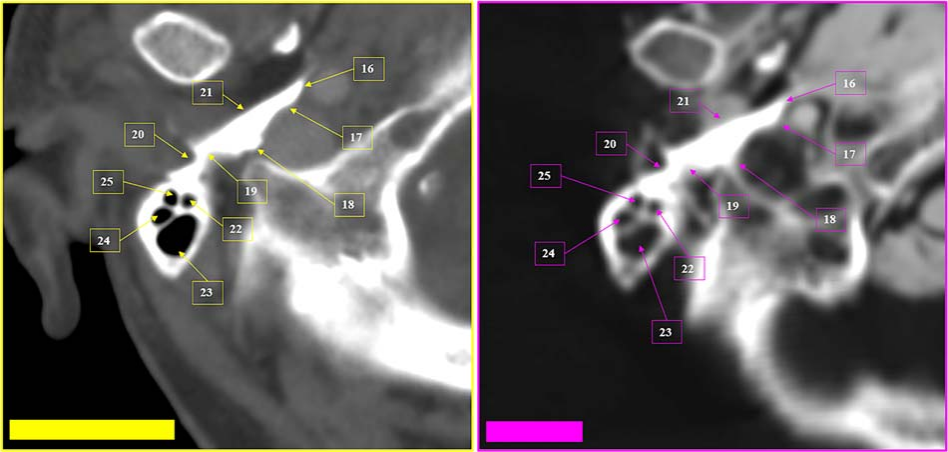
Coinciding morphology details and the arrangement of right mastoid cells in the antemortem (left) and postmortem (right) CT scans in axial sections.
